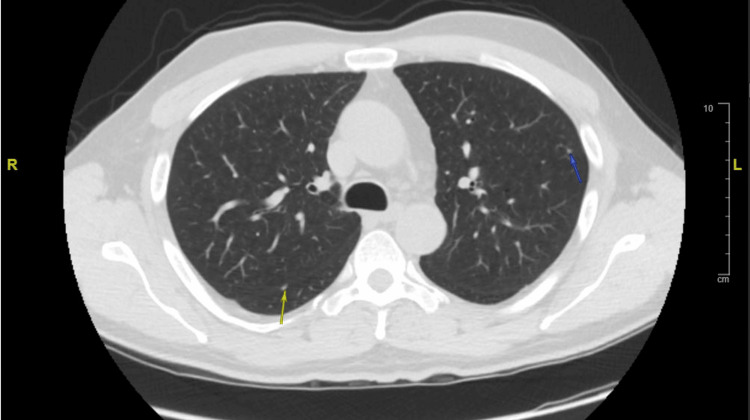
CT chest from 2022 showing a 3 mm left upper lobe nodule (blue arrow) and a 5 mm perifissural right lower lobe nodule (yellow arrow). Not pictured are a 6 mm perifissural right lower lobe nodule and a large left lower lobe consolidation, which were also present.
